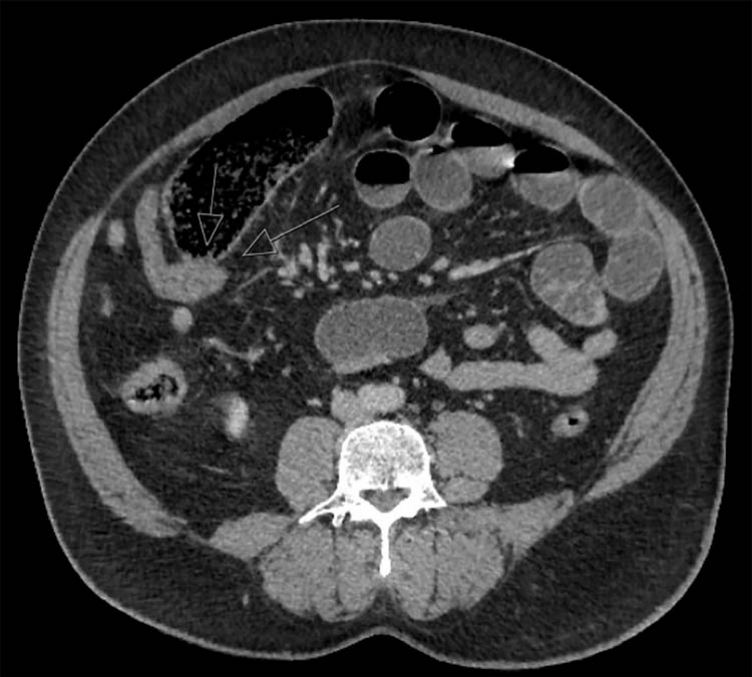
Case 2 CT abdomen axial view showing a distal SBO.
